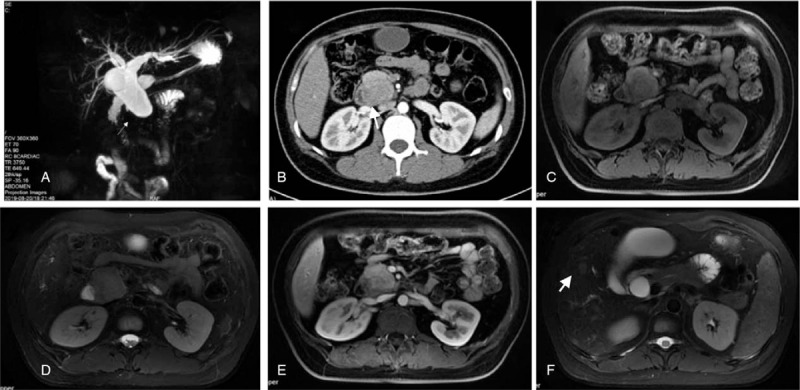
A: Magnetic resonance cholangiopancreatography showed dilated intrahepatic and extrahepatic bile ducts, enlarged gallbladder and tumor mass (white arrow); B: CT image of tumor (white arrow); C (T1 image) and D (T2 image): The mass abnormal signal of the pancreatic head of the lower common bile duct was about 44 mm × 41 mm, showing slightly longer T1 and longer T2 signal; E: The enhanced enhancement was slightly weaker than that of the surrounding pancreatic tissue; F: Liver lesion (white arrow).
